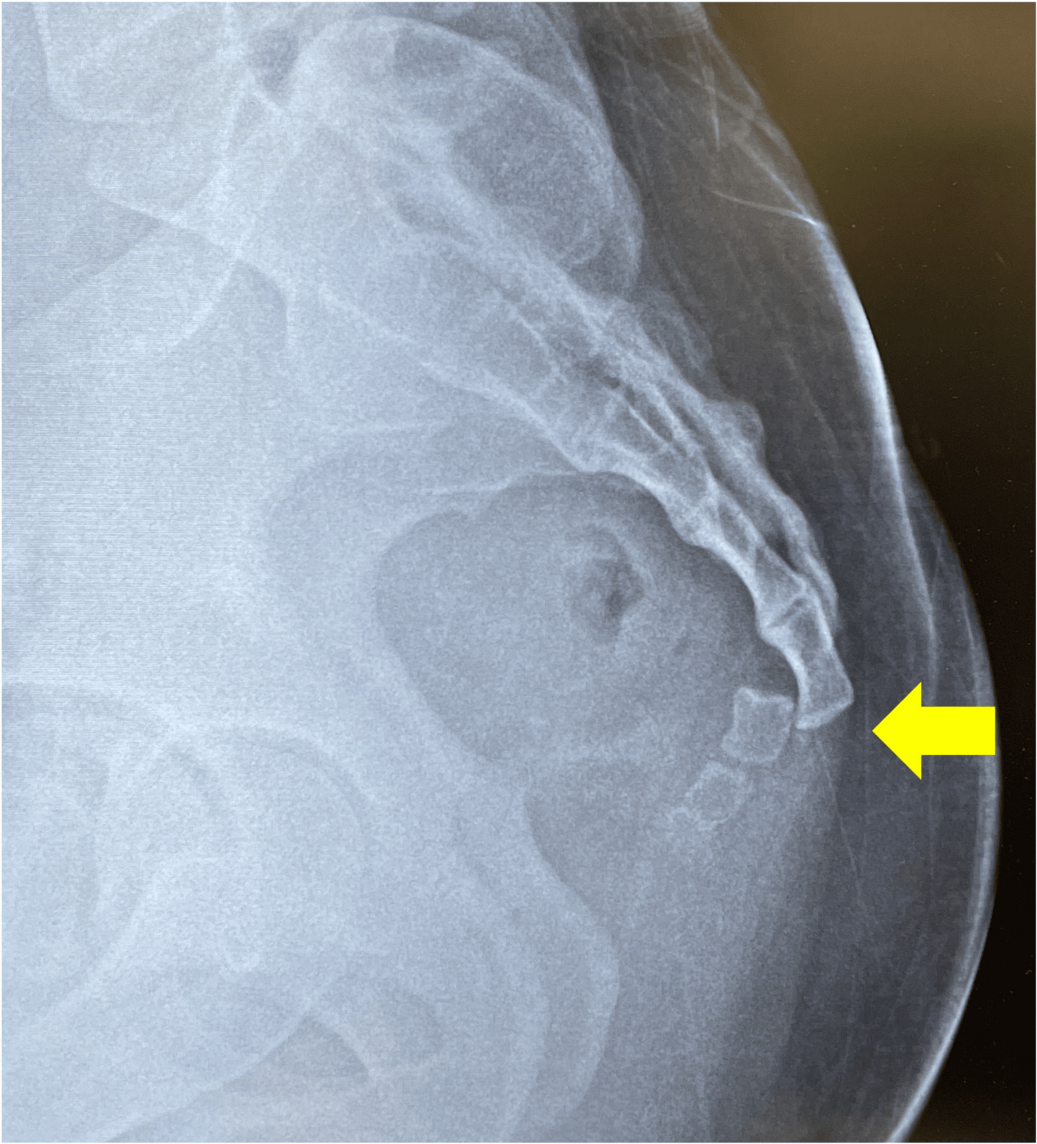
Imaging at the time of injury. Lateral radiograph showing anterior dislocation of the caudal vertebrae (arrow).
